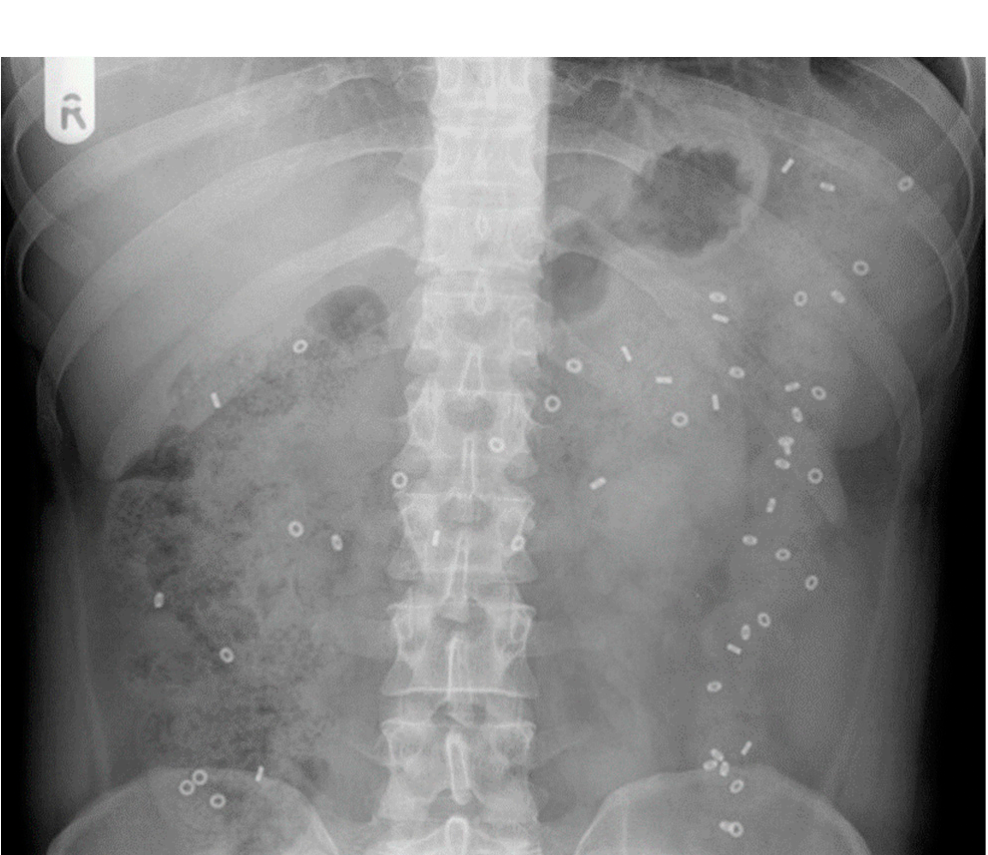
A plain abdominal X-ray image of radiopaque markers (ROMs) in the colon of a patient with constipation.
